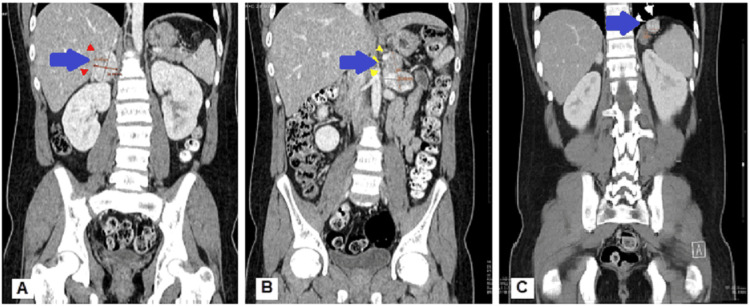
Abdominal CT images (coronal section) The coronal section of abdominal computed tomography shows a 42 x 34 mm right adrenal mass that is lobulated with exophytic enhancement, central hypodensities, and suggestive necrosis (A), a 43 x 27 mm left adrenal mass that is lobulated with exophytic enhancement and central hypodensities, suggesting necrosis (B), and a left-sided extra-adrenal lesion (C).
